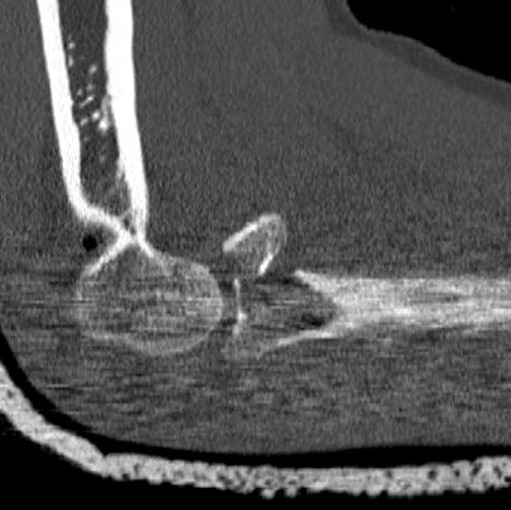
Same patient: preoperative CT scans, demonstrating the Mason Type III radial head fracture. No associated bony injuries could be detected.
